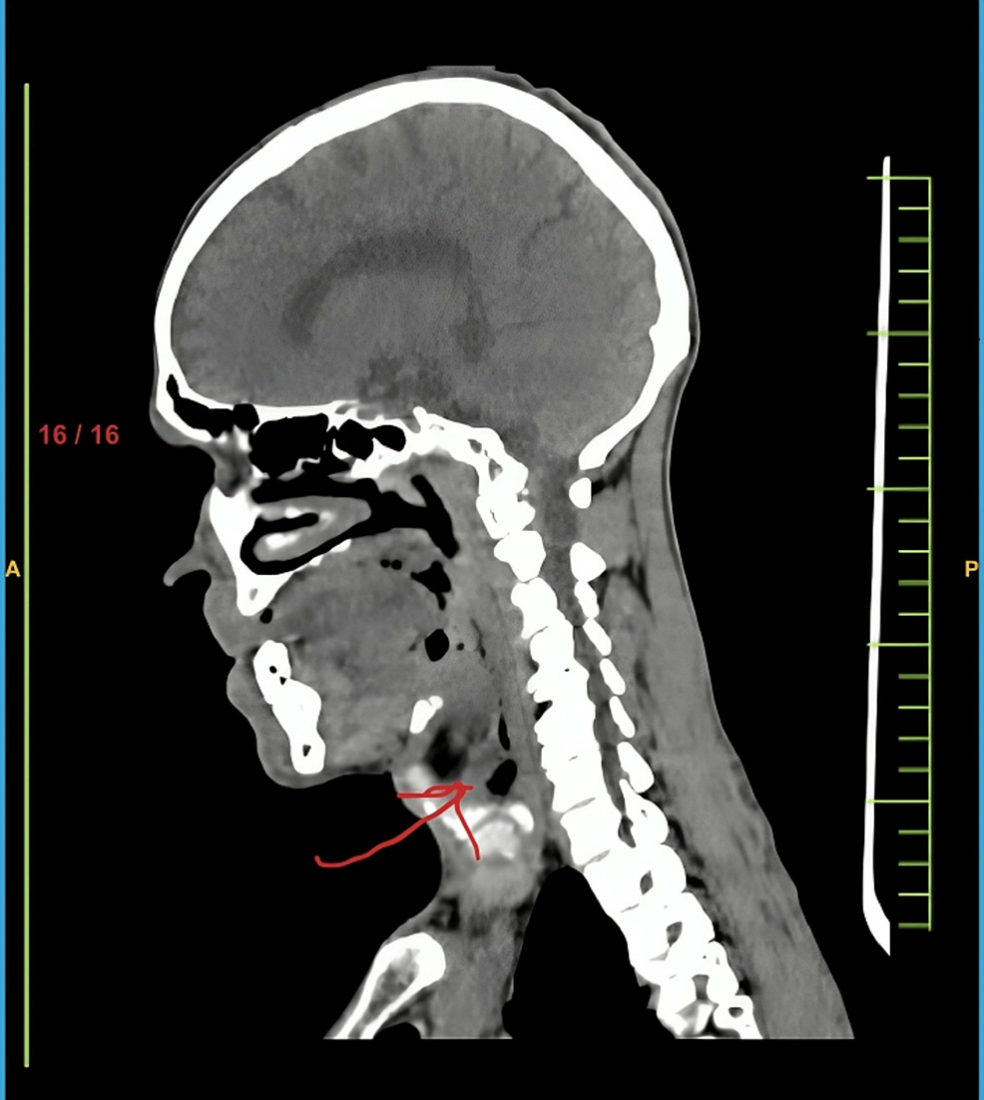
Contrast-enhanced CT scan showing a heterogeneously enhancing lesion (marked by a red arrow) in the supraglottic region
